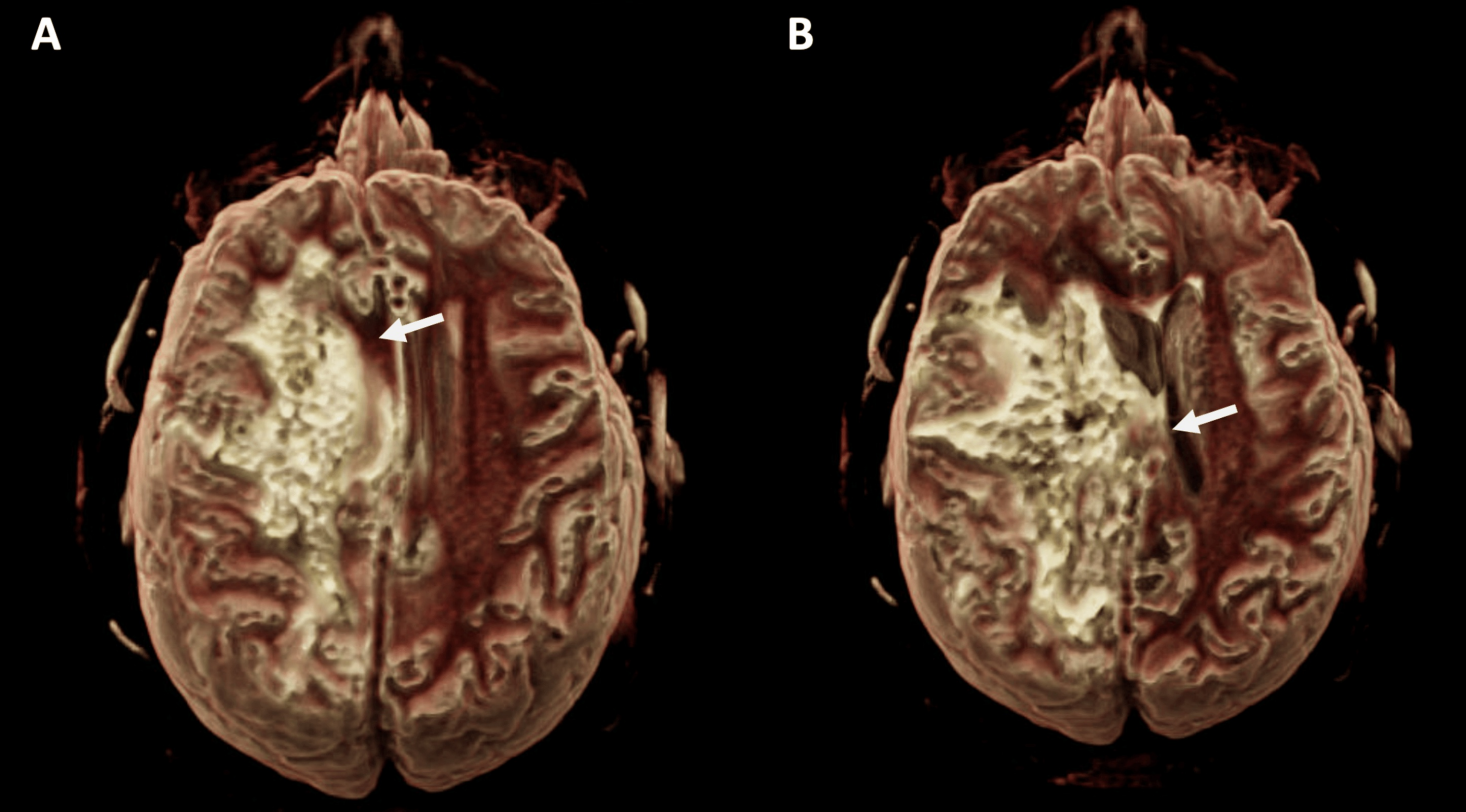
Cinematic-rendered volumetric T2 FLAIR images (A and B) show subcortical white matter signal abnormalities in the left cerebral hemisphere (arrows). The patient is seen from the back
